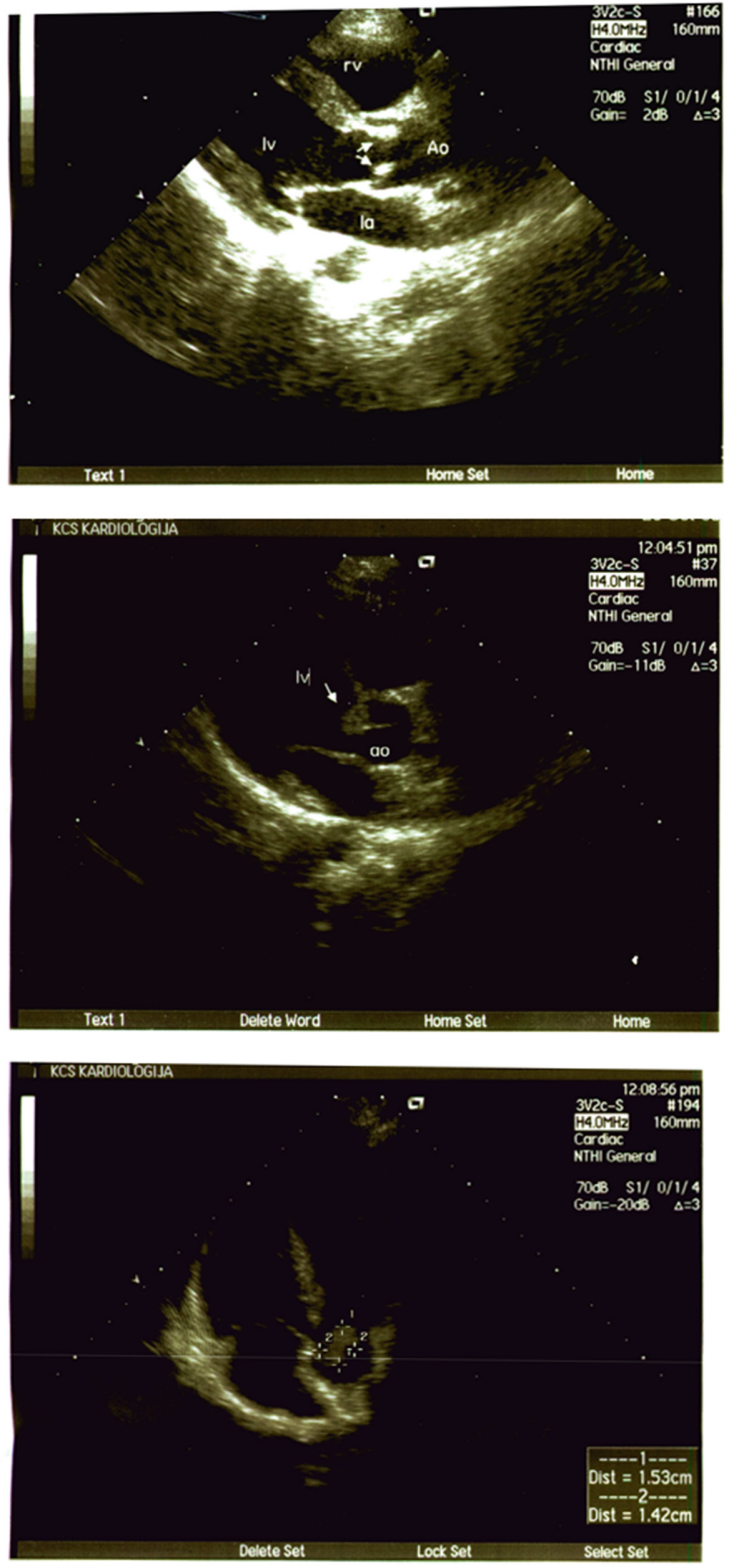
Transthoracal echocardiografy in longitudinal and apical 4 chamber view vegetations on the aortic valves.
